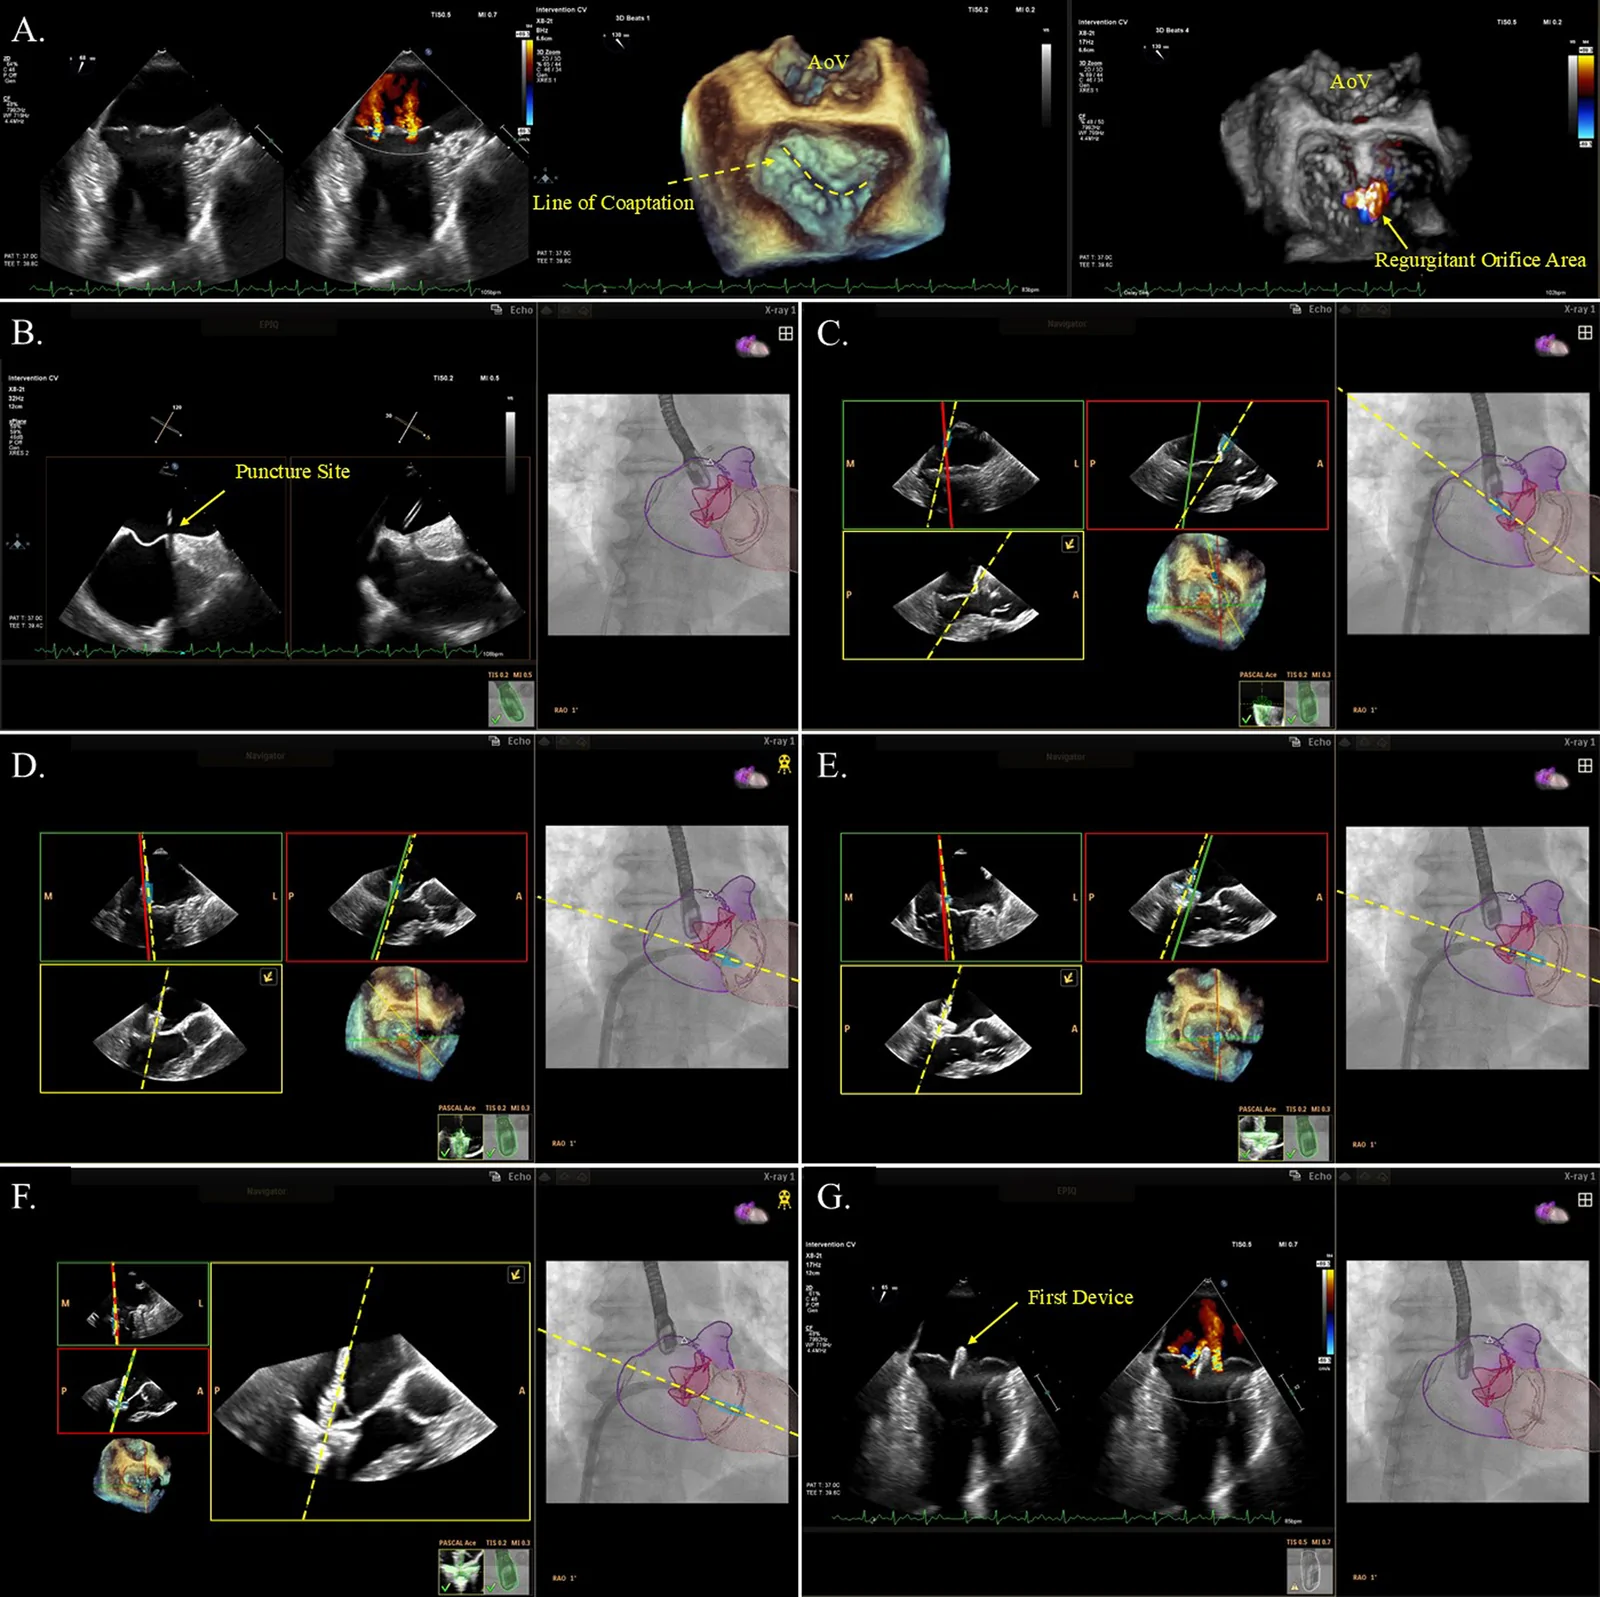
Stepwise echocardiographic guidance during mitral transcatheter edge-to-edge repair (M-TEER). A Baseline 2D transesophageal echocardiography (TEE) long-axis view and 3D TEE *en face* view demonstrating asymmetric P2–P3 prolapse with a broad regurgitant orifice. B The 2D TEE bicaval view demonstrating appropriate transseptal puncture height above the mitral coaptation plane. C–E DeviceGuide (EchoNavigator SmartVue, Philips Healthcare) displaying real-time multiplanar reconstruction (MPR) views with continuous visual indicators of device position, trajectory, and rotational orientation relative to the target zone defined at the coaptation line. F DeviceGuide with MPR and 3D TEE *en face* view confirming symmetric bilateral leaflet insertion and appropriate device depth prior to TEER device closure. G Postdeployment 2D TEE with color Doppler demonstrating a substantial but insufficient mitral regurgitation reduction to grade 2 +. AoV, aortic valve
